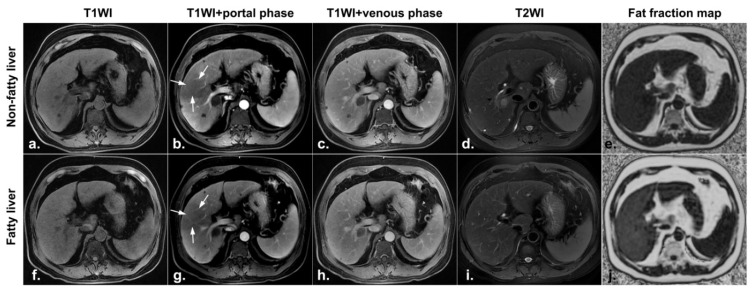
A 46-year-old male patient underwent interventional ablation of a small hepatocellular carcinoma. MRI images one month after radiofrequency ablation (a–e): no lesions were found on both T1W (a,f) and T2W (d,i); the fat fraction map showing no fat deposition in the liver (e); a contrast-enhanced image in the arterial phase showing hypoperfused foci(white arrows) in the liver (b); isointense signals in the portal venous phase (c). MRI images four months after radiofrequency ablation (f–j): a fat fraction map showing new fat deposition in the liver (j); a contrast-enhanced image in the arterial phase showing no obvious change in hypoperfused foci (white arrows) (g).
